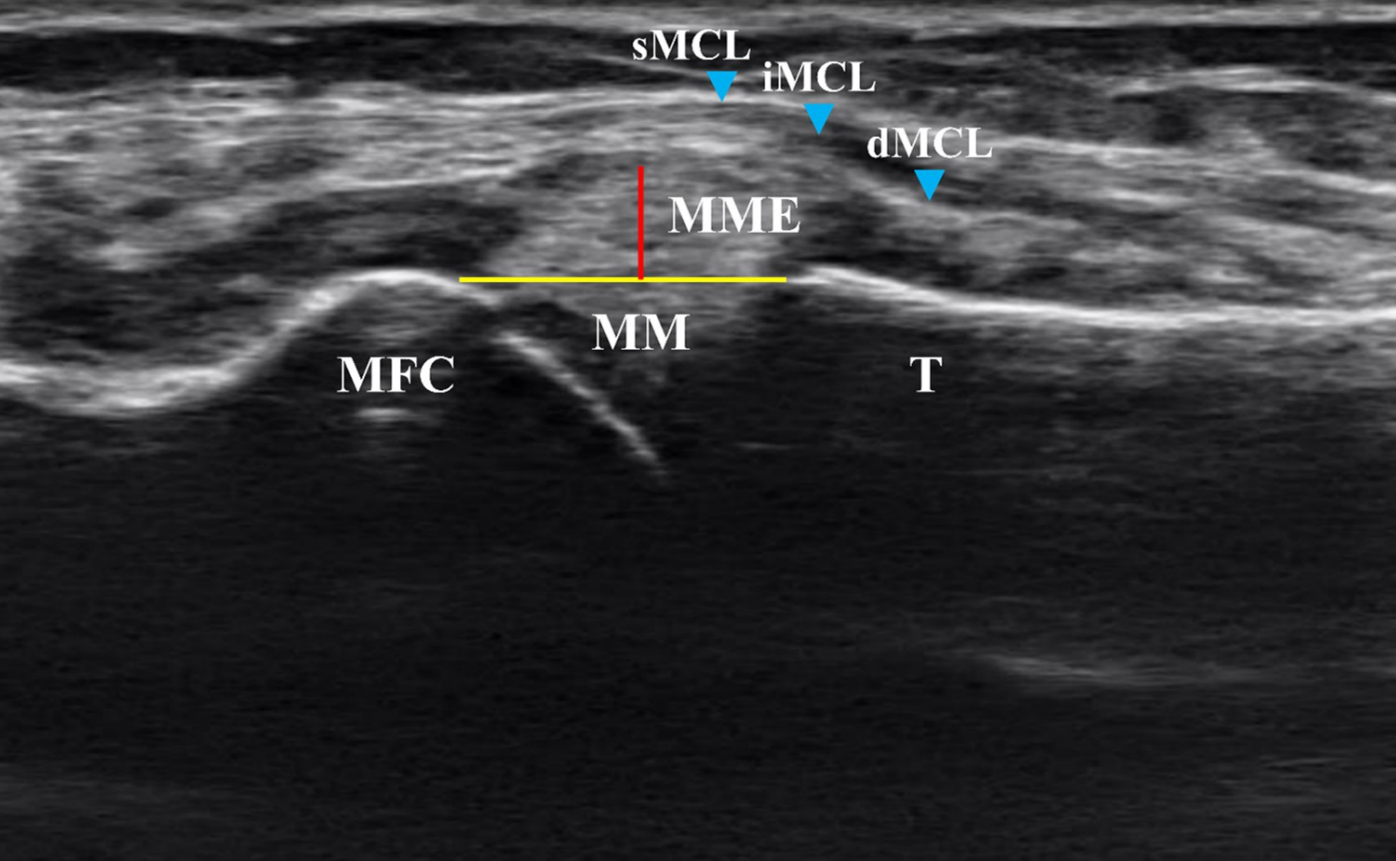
Definition of MME measurements. Longitudinal ultrasound images at the level of the MCL at the medial aspect of the knee joint. MME (red line) was measured as the distance between the outermost border of the medial meniscus and the line connecting the medial borders of the femur and tibia (yellow line). Abbreviations: sMCL (light blue arrowhead), superficial bundle of the medial collateral ligament (MCL); iMCL (light blue arrowhead), intermediate superficial bundle of the MCL; dMCL (light blue arrowhead), deep posterior bundle of the MCL; MME, medial meniscal extrusion; MM, medial meniscus; MFC, medial femoral condyle; T, tibia.
